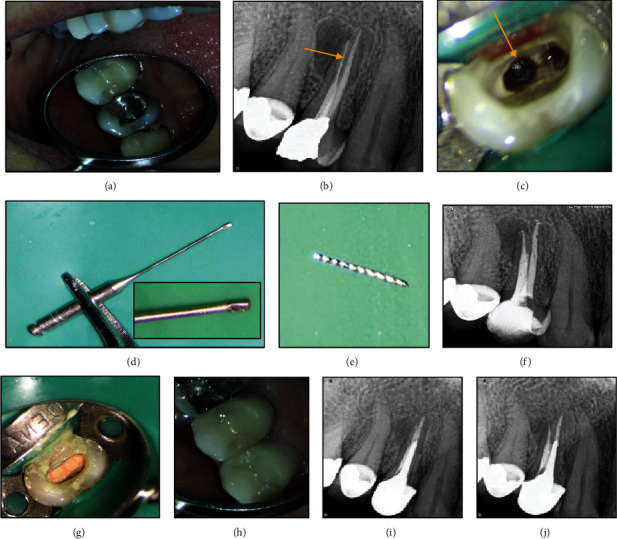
(a) Intraoral examination. (b) Radiographic examination showing periapical radiolucency and a broken instrument in the palatal canal. (c) Coronal portion of the broken file in the palatal canal was visualized under a DOM. (d) Modified Gates-Glidden drill. (e) Retrieved file fragment. (f, g) Canal preparation and obturation. (h, i) 6-month follow-up. (j) 2-year follow-up: evidence of healing of the apical radiolucency was seen.
